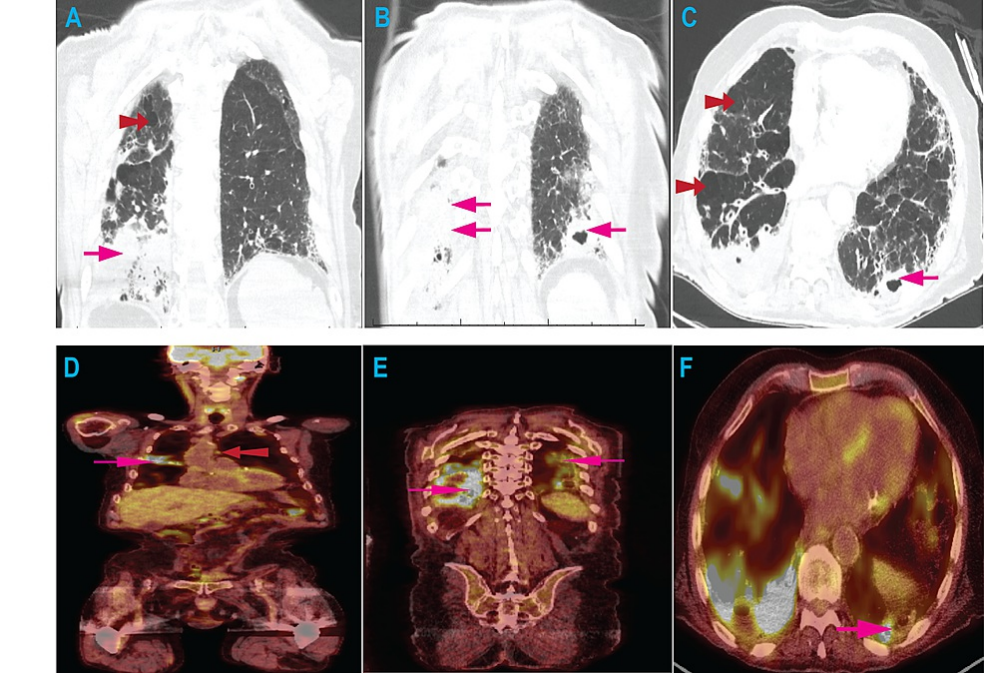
Radiologic findings A and B. CT scan chest (coronal view). Dense infiltrates (pink arrows) are present in a predominant posterior distribution, most pronounced in the right lower lobe. There are also several large bullae (red double arrowheads), consistent with centrilobular and paraseptal emphysematous changes. The infiltrates were not present on a CT scan two years earlier. C. CT scan chest (axial view). A 3.0 cm thick-walled cavitary lesion is seen in the left lower lobe base (pink arrow), also seen in panel B. D and E. 18-FDG PET scan (coronal view, anterior and posterior sections). There are intense streaks and patches of 18-FDG uptake (pink arrows) with maximum standardized uptake values (SUV) ranging from 29 to 45, significantly brighter than the physiologic uptake in the myocardium. Other regions within the infiltrate have minimal metabolic activity. The bilateral hilar and mediastinal lymph nodes have modestly increased uptake, SUV <5 (red double arrowhead). F. PET scan chest (axial view). There is significant metabolic activity in part of the rim of the cavitary lesion (pink arrow). FDG – fluorodeoxyglucose; PET – positron emission tomography
